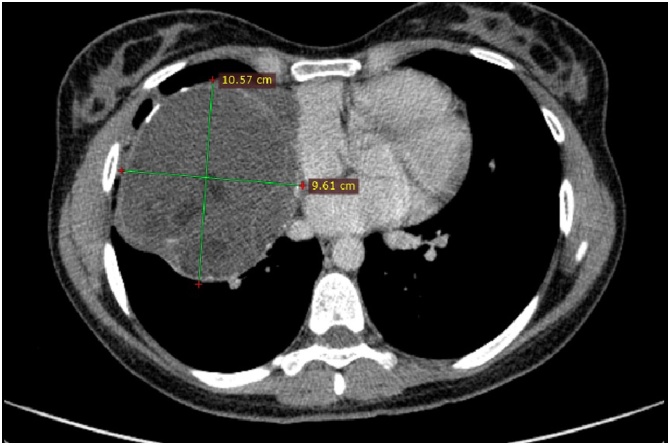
Axial contrast-enhanced CT image at the level of the pulmonary artery trunk shows a round, anterior mediastinal mass with heterogenous content.
